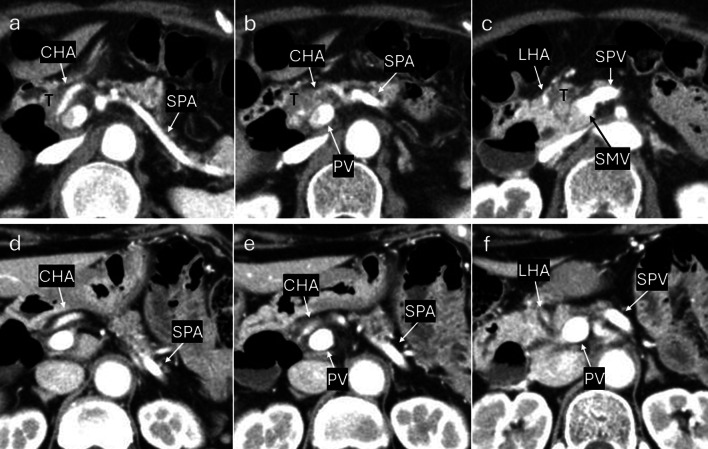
Multidetector row computed tomography (MDCT) scan findings of a pancreatic adenosquamous carcinoma. a–c Contrast-enhanced MDCT scan findings before conversion-intended chemotherapy. The tumor had invaded the common and left hepatic arteries and contacted more than half the circumference of the portal vein. d–f Contrast-enhanced MDCT scan findings after conversion-intended chemotherapy. The tumor in the pancreatic head shrank to release the involvement of the common hepatic and left hepatic artery. The contact with the tumor to the portal vein became less than half the circumference of this vein. T, tumor; CHA, common hepatic artery; SPA, splenic artery; PV, portal vein; LHA, left hepatic artery; SPV, splenic vein; and SMV, superior mesenteric vein
